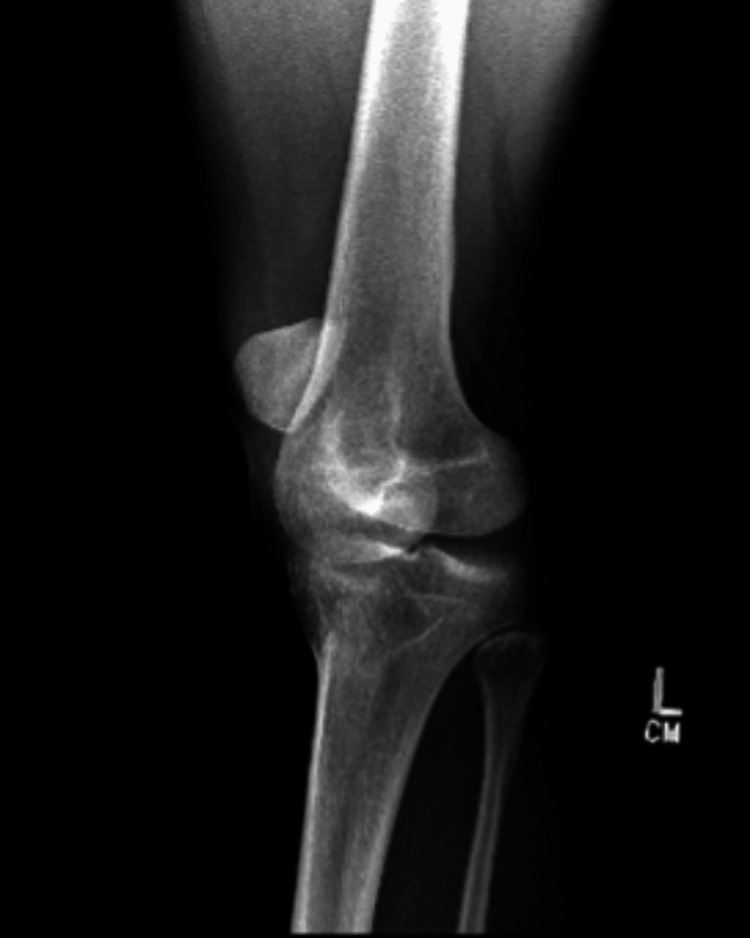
X-ray (oblique view) post-reduction
